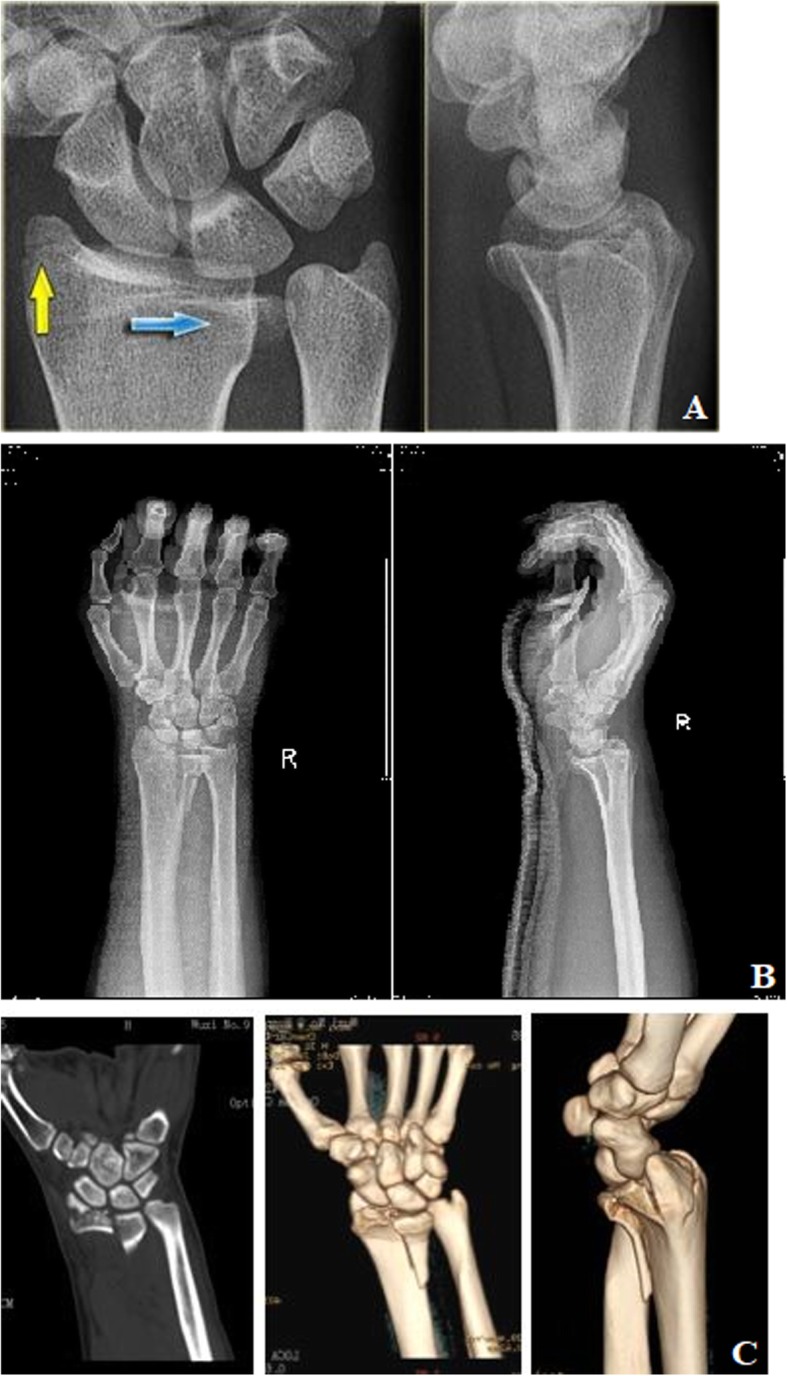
PA X-ray in example 1 (a), PA X-ray in example 2 (b), and CT scans in example 2 (c) of articular surface radius column fracture
